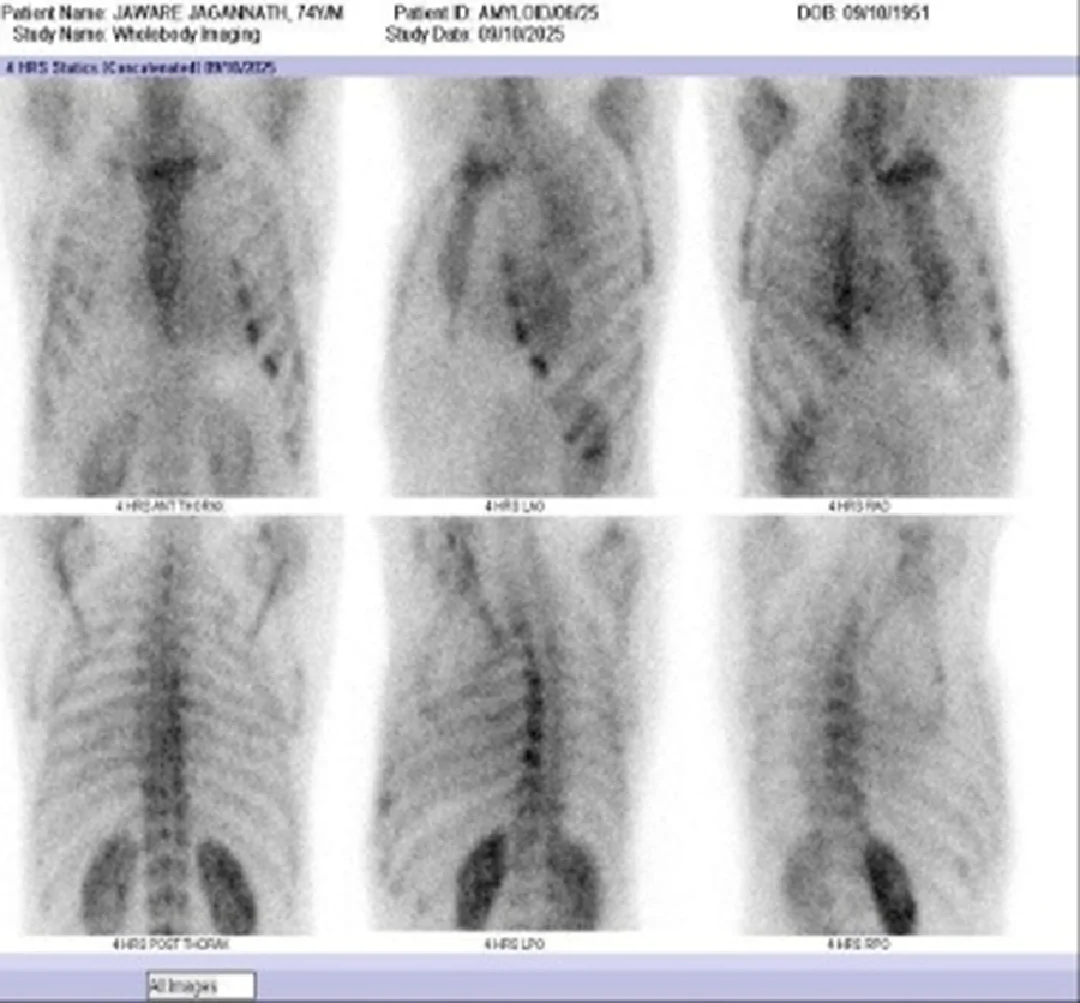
Delayed (4-hour) planar 99mTc-pyrophosphate scintigraphy. Anterior, left anterior oblique (LAO), right anterior oblique (RAO), posterior, left posterior oblique (LPO), and right posterior oblique (RPO) thoracic images demonstrate persistent diffuse myocardial radiotracer uptake with reduced blood-pool activity.
